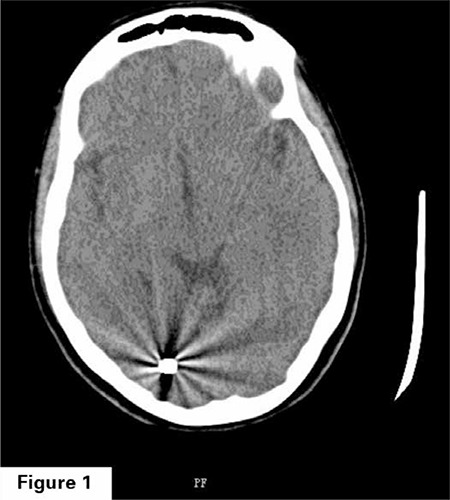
A bullet with 9x6 mm can be seen in the left posterior parieto-occipital area in the cranial CT horizontal cross-section
